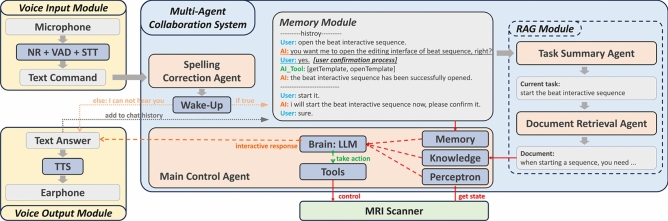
Voice-based touchless control system for MRI scanners. Our voice-controlled system consists of three primary modules: a voice input module for capturing and processing spoken commands, a multi-agent collaboration system (MACS) that interprets and executes commands to control the MRI scanner, and a voice output module for providing auditory feedback. ’Interactive response’ represents three actions – report action result, request confirmation, and request for further explanation.
